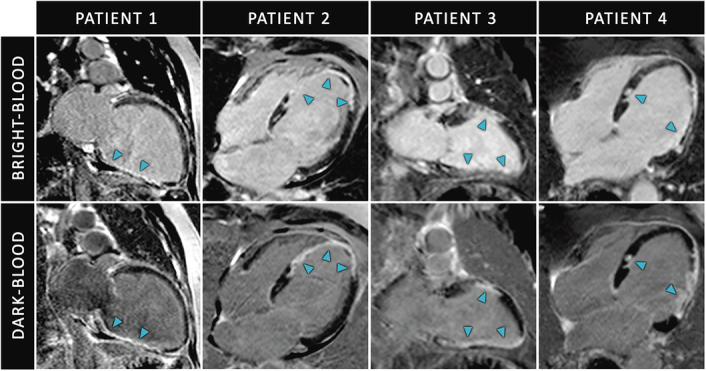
Conventional bright-blood and dark-blood late gadolinium enhancement (LGE) cardiac magnetic resonance images. The blue arrowheads indicate ischemic scars.
